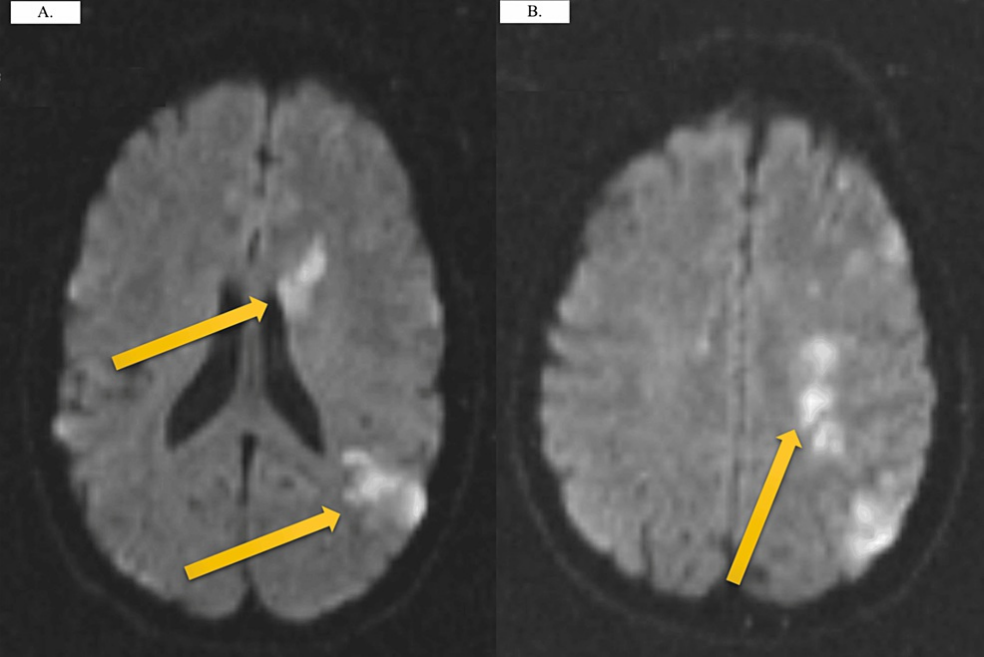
MRI demonstrating (A) acute left MCA, ACA, and watershed infarct (B) restricted diffusion within the distribution of the left MCA. Yellow arrows indicate regions of infarct. MCA = middle cerebral artery; ACA = anterior cerebral artery
